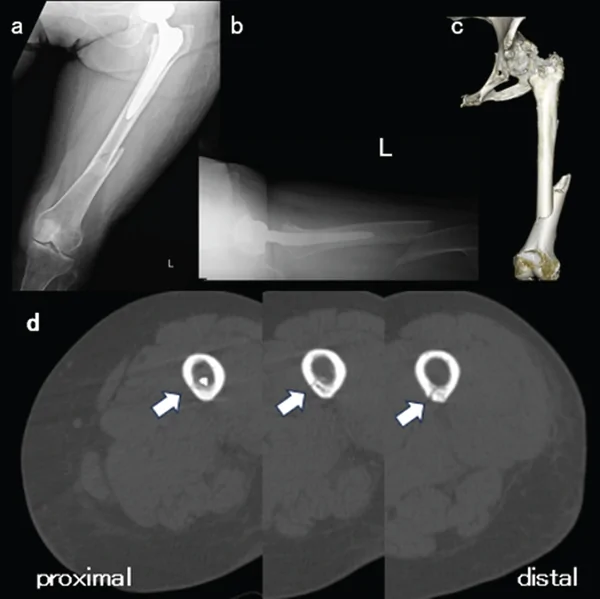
Images obtained 9 days after total hip arthroplasty when the patient developed acute severe pain in the left thigh when climbing stairs. (a) Anteroposterior radiograph showing an oblique periprosthetic femoral fracture distal to the femoral stem, which was classified as Vancouver type C (postoperative). (b) Cross‐table lateral radiograph confirming fracture displacement and cortical disruption. (c) Three‐dimensional computed tomography reconstruction image demonstrating an oblique Vancouver type C (postoperative) fracture without evidence of implant loosening. (d) Axial computed tomography images showing a fracture posterior to the femur at the level of the distal tip of the stem. The white arrows indicate the fracture site.
